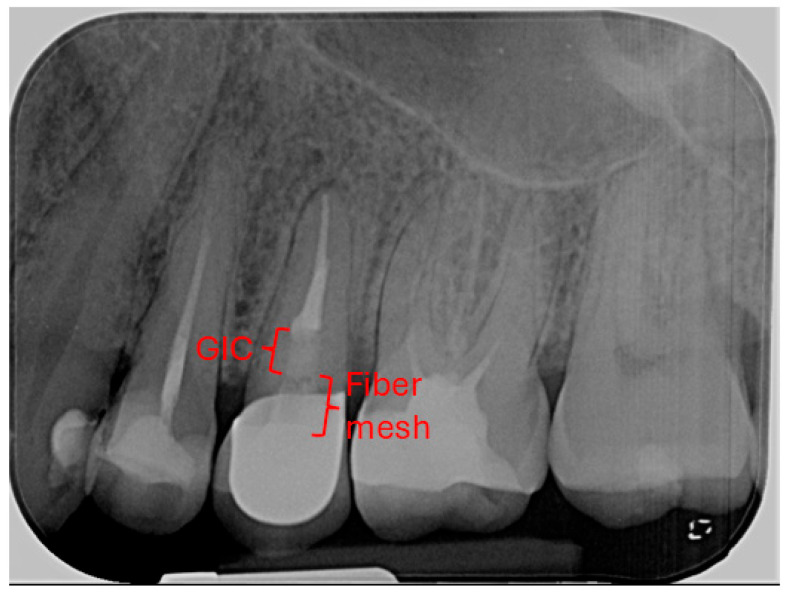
A 2.5-year follow-up periapical radiograph of tooth 25. The GIC base and the fiber mesh component of the coronal restoration are indicated on the radiograph.
